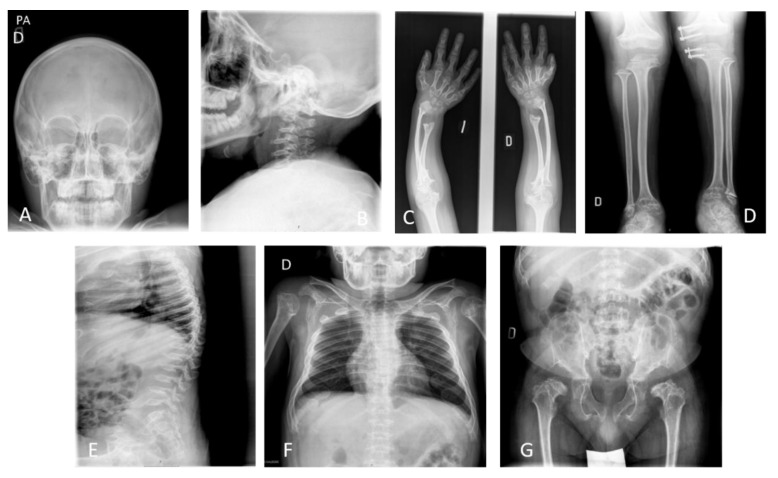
X-ray images of a 17-year-old male patient with MPS IVA. Images show skeletal dysplasia (dysostosis multiplex); (A) incomplete ossification and prominent forehead, (B) incomplete ossification in odontoid process and subluxation of the atlas secondary to odontoid hypoplasia with platyspondyly of cervical vertebrae, (C) cortical thinning and mild widening of the diaphysis of the humerus and tilting of the radial epiphysis towards the ulna producing curvature, (D) genu valgum with cortical thinning of tibia and fibula, (E) the accentuated dorsal thoracolumbar kypholordosis with the advanced platyspondyly, irregularity, and anterior beaking of vertebral bodies characteristic of MPS IVA and flared ribs, (F) abnormal thoracic cage, pectus carinatum and scoliosis with oar shaped ribs: the ribs are wide anteriorly and laterally and overconstricted in their paravertebral portions, (G) spondyloepiphyseal dysplastic femoral heads and oblique acetabular roof with coxa valgus deformity and flared iliac wings.
